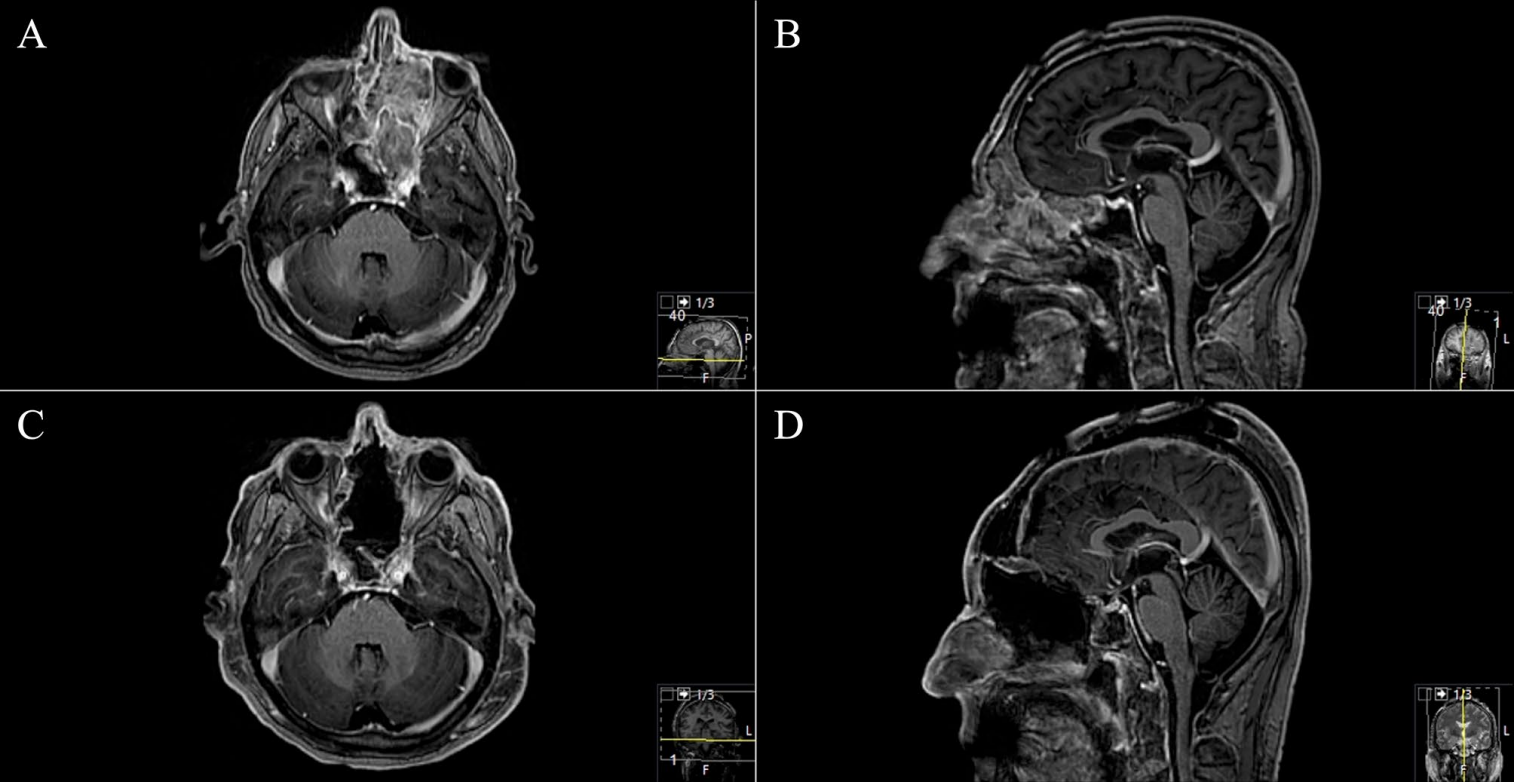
Exemplary case 2: a 70-year-old male patient presented with recurrent left eye inflammations and swelling over medial canthus. Ophthalmic examination revealed obstructed nasolacrimal ductus. (A,B) Preoperative T1-weighted gadolinium-enhanced MR imaging showing sinonasal and left orbit-infiltrating tumor. Ethmoidal and sphenoid sinus are completely infiltrating. Left bulbus is suppressed. (C,D) Postoperative MR control showing successful resection. Orbit, sphenoid and tuberculum sellae reconstructions were performed with split-bone graft, galea-periosteum flap and fibrin glue. Pathologic findings revealed metastasis of malign melanoma. The patient underwent endoscopic transnasal, sublabial reresection in the subsequent course.
